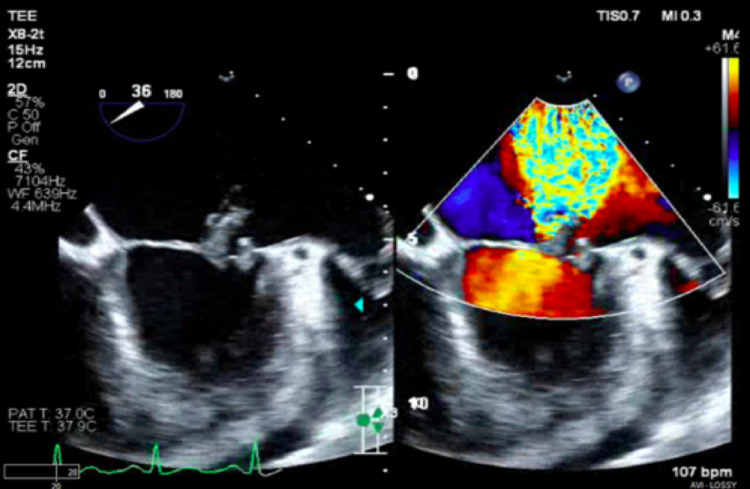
Transesophageal echocardiogram (TEE) image depicting moderate-to-severe mitral regurgitation with the jet bouncing off the back wall of the left atrium
